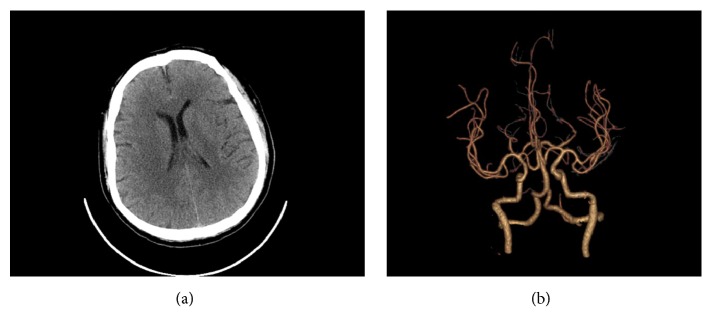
Initially, (a) a noncontrast computed tomography (CT) and (b) a computed tomography angiography (CTA) of the head performed on at the time of the admission showed no abnormalities and vascular malformations.
